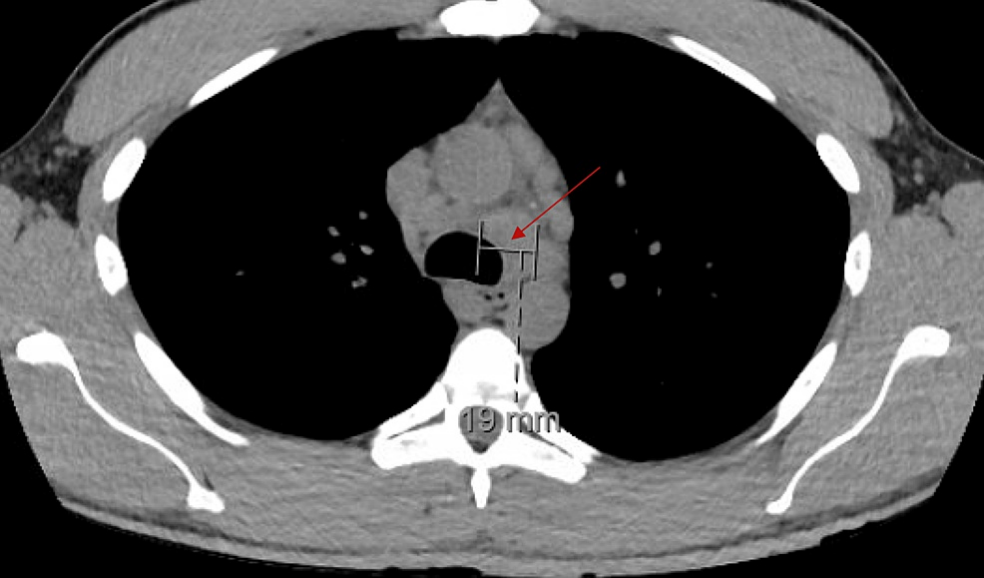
CT Thorax without contrast: mediastinal adenopathy with largest lymph node measuring 19 mm.
